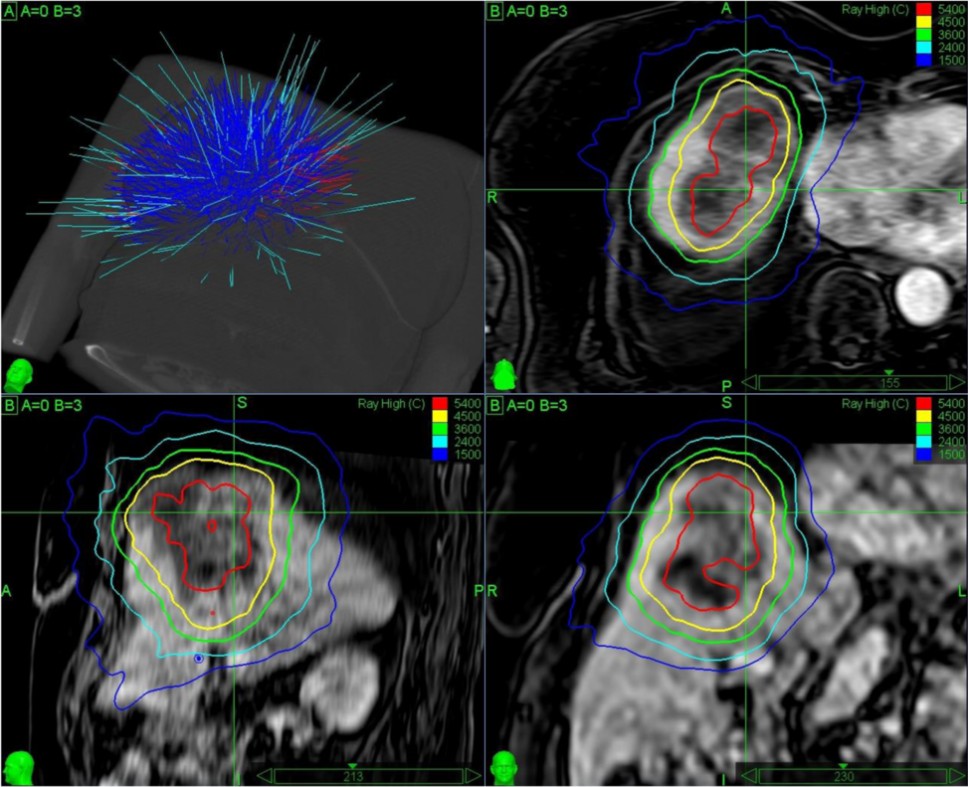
CyberKnife treatment plan of a 226 cc metastasis in the upper liver overlaid on contrast enhanced T1-weighted MRI in axial (*upper right*), sagittal (*lower left*), and coronal (lower right) view. The prescription dose to the planning target volume was lowered to 3 × 12 Gy (BED_10_ = 79.2 Gy_10_) to the 60 % isodose due to the size and critical organ constraints (i.e., the heart). The mean gross tumor volume dose was optimized to 140 Gy_10_. Twenty-eight months after treatment, the patient was alive and had complete response with no further development of metastases in the meantime. Upper left: Planning CT with final CyberKnife treatment beams (cyan) from the initially generated beam assortment (*blue*/*red*)
